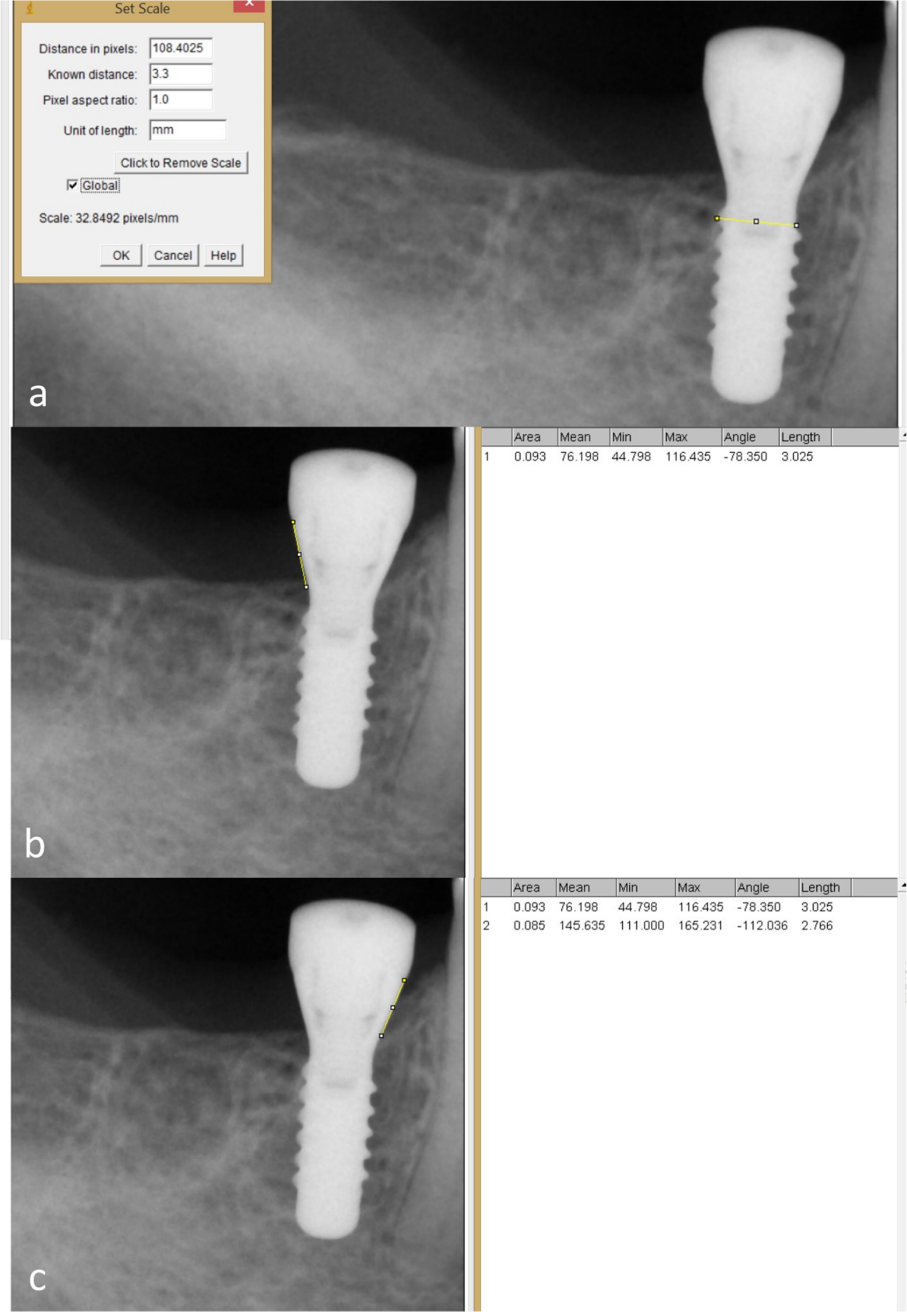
Radiographic images disclosing the principle for MBL assessment at visit 7. a Adjustment of distortion coefficient. b Distal aspect of the implant. c Mesial aspect of the implant. For further measurements, the mean values from distal and mesial aspects were calculated
